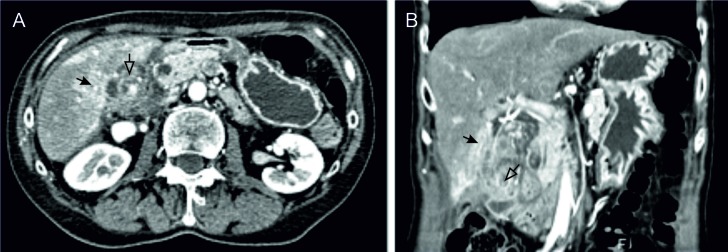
Abdominal imaging findings A. Axial CT plane; and B. Coronal CT plane, showing an irregular heterogeneous mass occupying the gallbladder fossa (hollow arrow) with the involvement of the adjacent liver segments (black arrow) and surrounding tissues. CT: computed tomography
